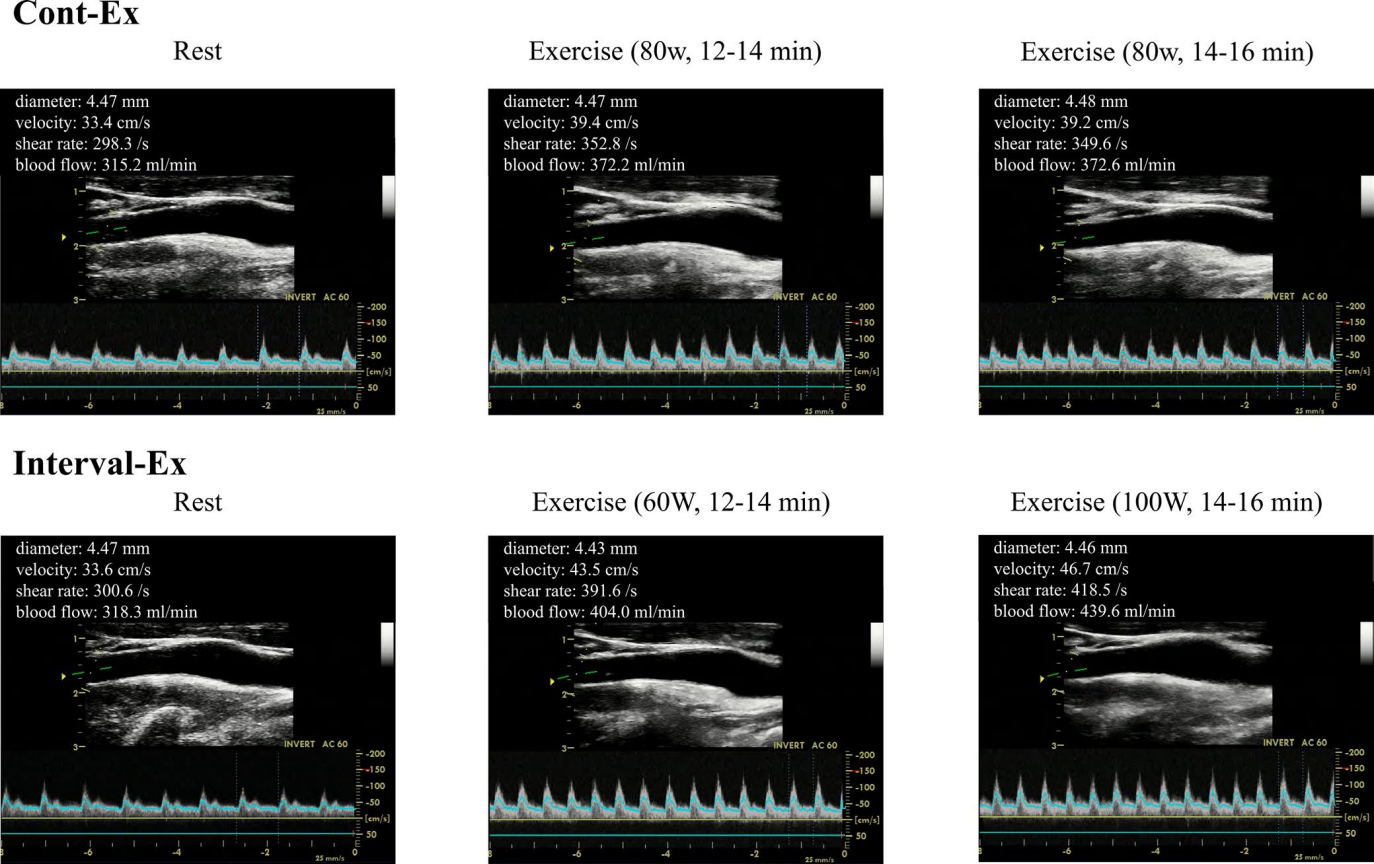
Internal carotid artery image and cerebrovascular parameters using Doppler ultrasonography
